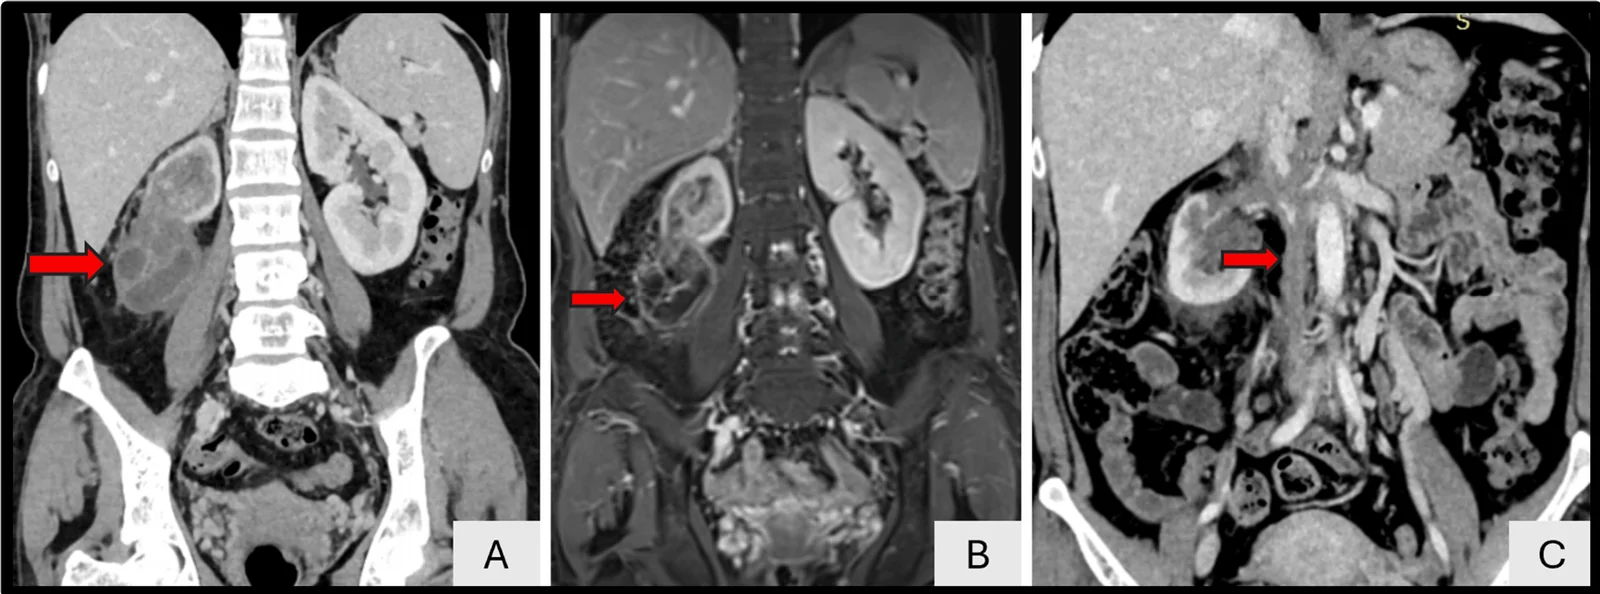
Post-treatment imaging demonstrating excellent response to systemic chemotherapy A: Coronal reconstructed cortico-medullary phase of CT urography image shows marked interval reduction in the size of the primary lesion following systemic chemotherapy with a cyclophosphamide and adriamycin-based regimen (red arrow). B: Coronal reconstructed post-contrast fat-suppressed T1-weighted MRI demonstrates significant regression of the lesion with an increase in cystic and necrotic components (red arrow). C: Coronal reconstructed corticomedullary CT urography image shows a consistent IVC tumor thrombus (red arrow) IVC: Inferior vena cava
